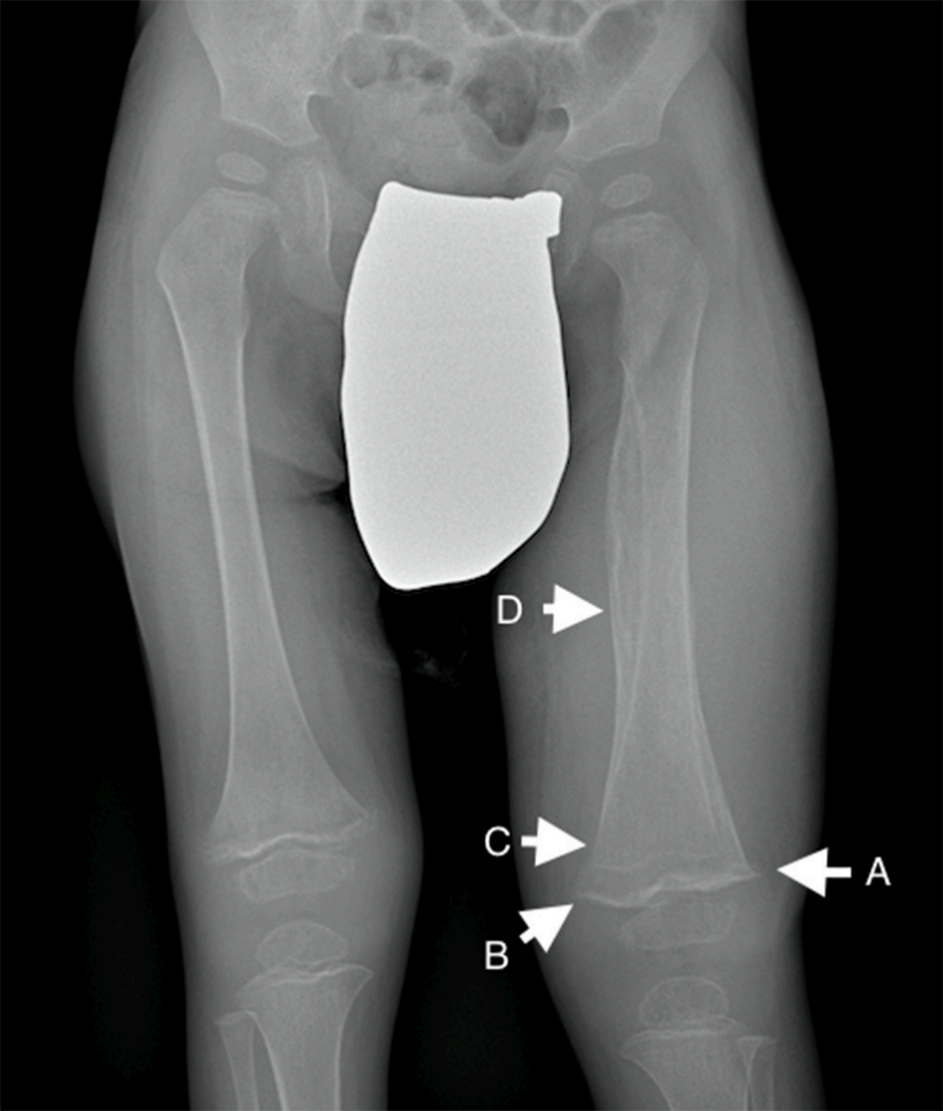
Lower limb X-Ray showing generalized osteopenia, metaphyseal spurs with concomitant cupping of the metaphysis (“Pelken spurs”, arrow A), lucent metaphyseal bands (“Trümmerfeld zone”; arrow B) and dense metaphyseal line (“Frankel's line”; arrow C). Extended periosteal reaction could also be documented (arrow D).
